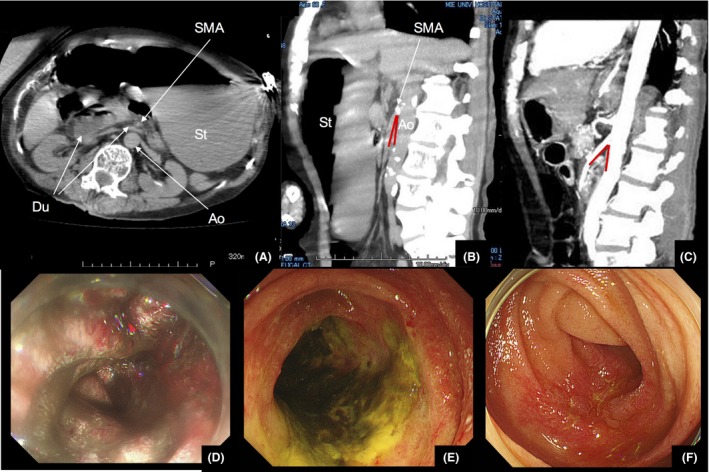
Computed tomography (CT) and endoscopic images of a 60‐year‐old woman with reversible acute mucosal gangrene accompanying septic shock that resulted from superior mesenteric artery (SMA) syndrome. A, CT axial section showing compression of the third part of the duodenum between the SMA and abdominal aorta (Ao), with proximal duodenal and gastric dilatation. B, Sagittal multiplanar reconstruction contrast‐enhanced CT showing the SMA arising from the aorta and forming a narrow aortomesenteric angle (AMA) (red lines) of 17° (in normal condition, 45–60°). C, Contrast CT carried out after gastric decompression showed the improvement of AMA (red lines) at 42° and revealed no mesenteric artery occlusion or mucosal ischemia. D, Emergency upper endoscopy suggested acute ischemia that resulted in superficial membranous gangrene; however, there was no evidence of transmural necrosis. E, F, Repeated upper endoscopy showed gradual improvement of the mucosal gangrene. Images in panels (E) and (F) were obtained on day 10 and 21, respectively. Du, duodenum; St, stomach.
